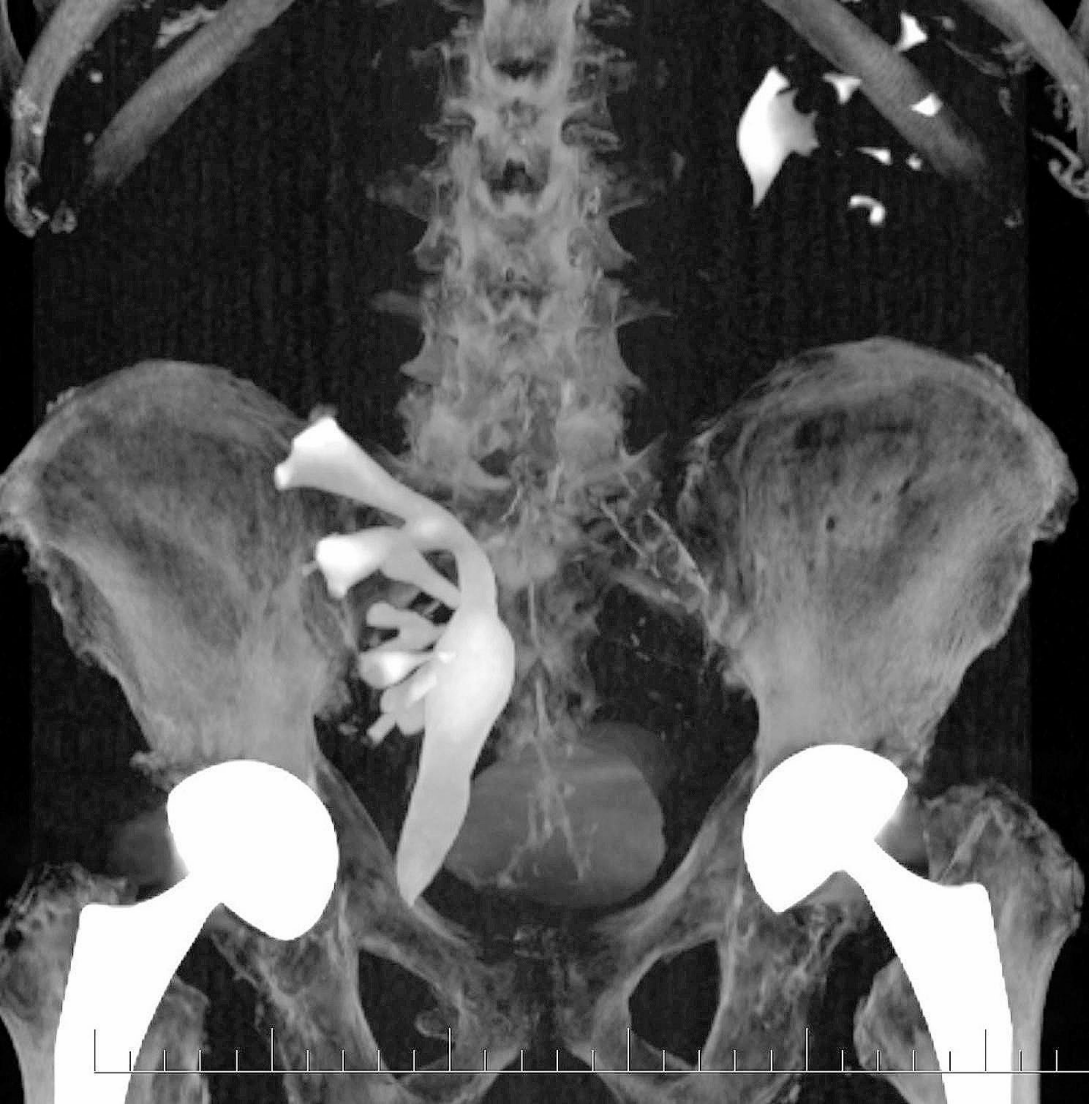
Reconstructed CT urography showing normal position of the left kodney, and ectopic position of the right kidney with urostasis
